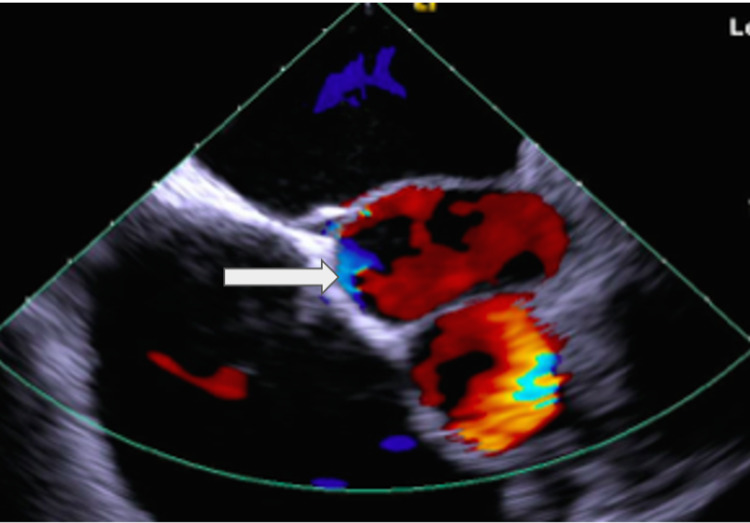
A transesophageal echocardiogram (mid-esophageal short-axis view) shows an abnormal structure (4 x 4 cm) adjacent to the aorta (white arrow).
